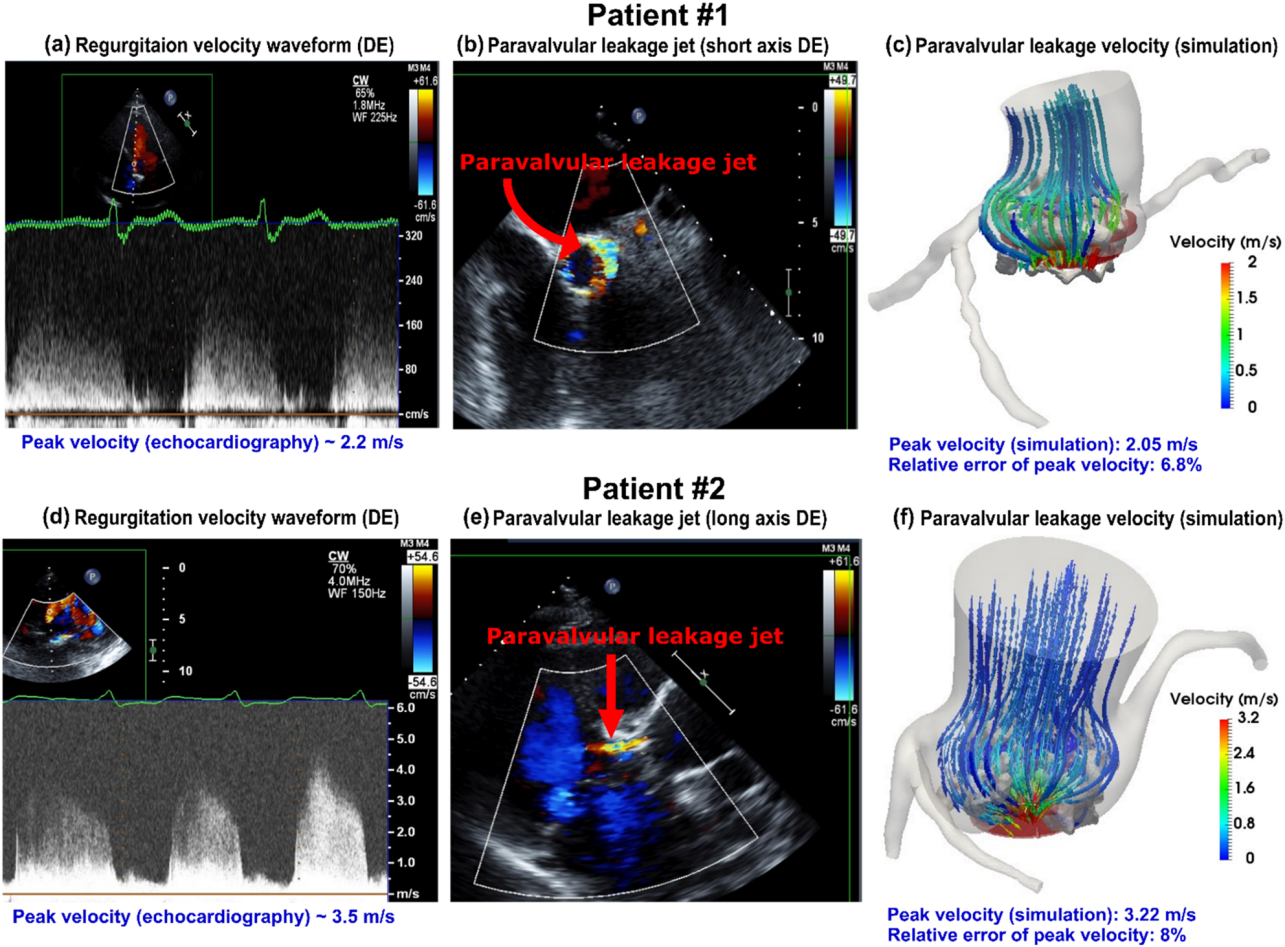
Validation: Doppler-based LPM and FSI framework versus patients Doppler echocardiography data. (a) & (d) Regurgitant flow waveform during diastole in patients #1 and #2; (b) Parasternal short axis view of PVL during diastole in patient #1; (e) Parasternal long axis view of PVL during diastole in patient #2; (c) & (f) PVL flow velocity streamlines during diastole in patients #1 and #2.
